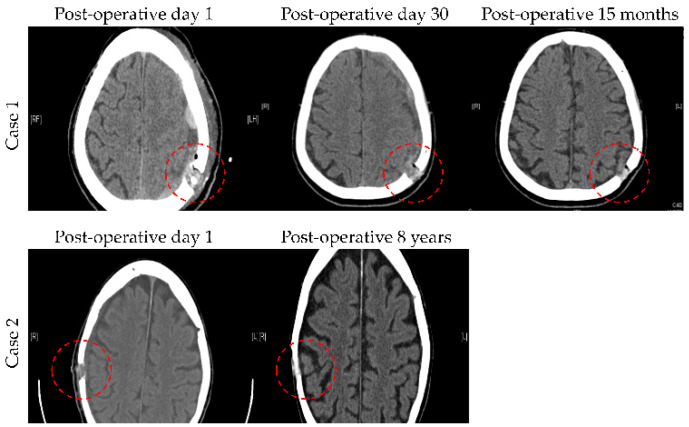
Two cases of serial postoperative computed tomography images demonstrating the bone ingrowth sufficiently covering the burrhole defect at varying stages of implantation over time. Reprinted/adapted with permission from Ref. [16]. 2020, Future Medicine, *Journal of 3D Printing in Medicine*.
